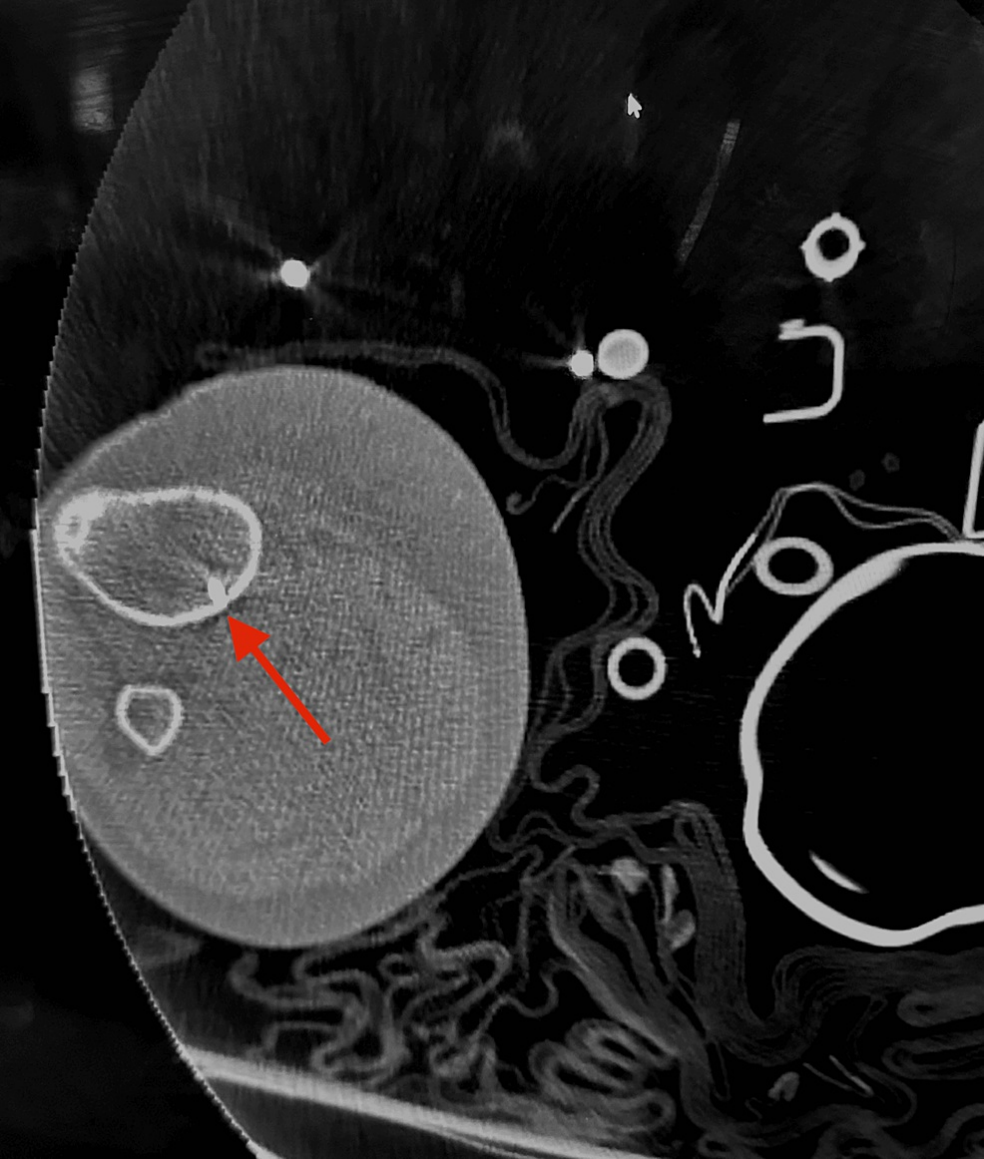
An IO catheter is seen extending into the proximal tibial diaphysis with a tip protruding through the posterior cortex (arrow) on CT of the right lower extremity. IO: Intraosseous
